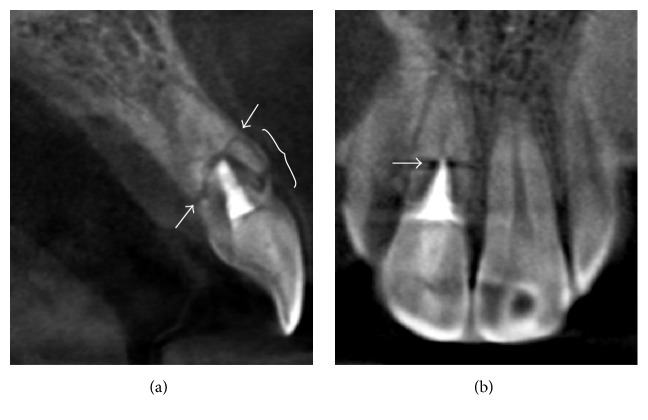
CBCT images (June 2015). (a) The sagittal CBCT slice of tooth 11 reveals a comminuted fracture on the labial aspect within the cervical third of the root (brace). A complete fracture line was found running obliquely from the middle third on the facial aspect through the cervical third on the palatal aspect (arrows). (b) The coronal CBCT slice reveals a horizontal root fracture in the middle third of the root without interposition of hard tissue (arrow).
